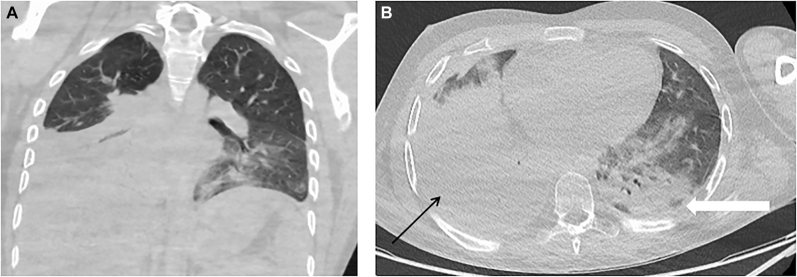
CT scan of the thorax upon admission showing signs of pleural effusion (thin black arrow) and inflammatory infiltrations (bold white arrow) which lead to global respiratory insufficiency.
